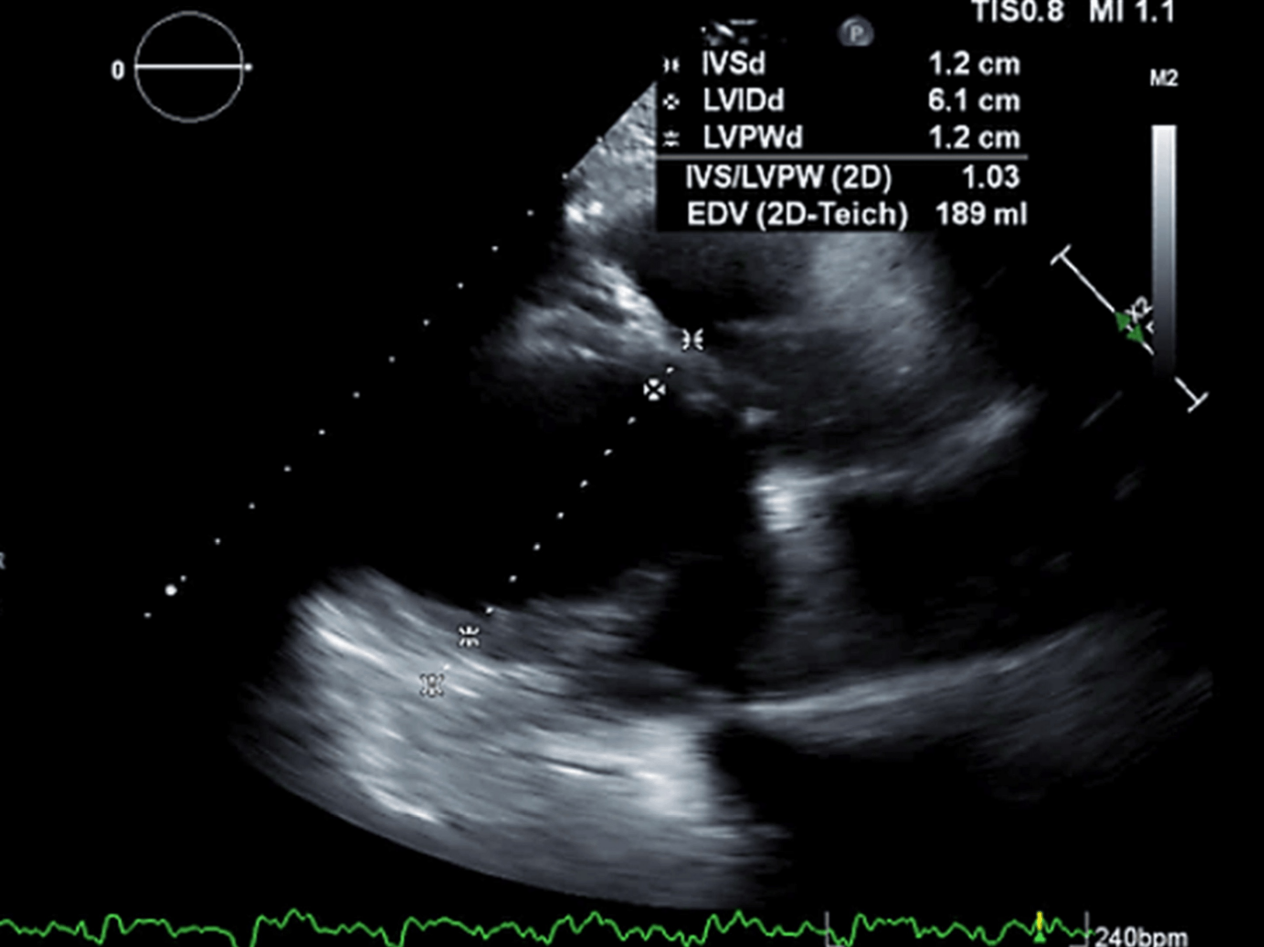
Transthoracic echocardiogram showing left ventricular diastolic dimension of 6.1 cm.
